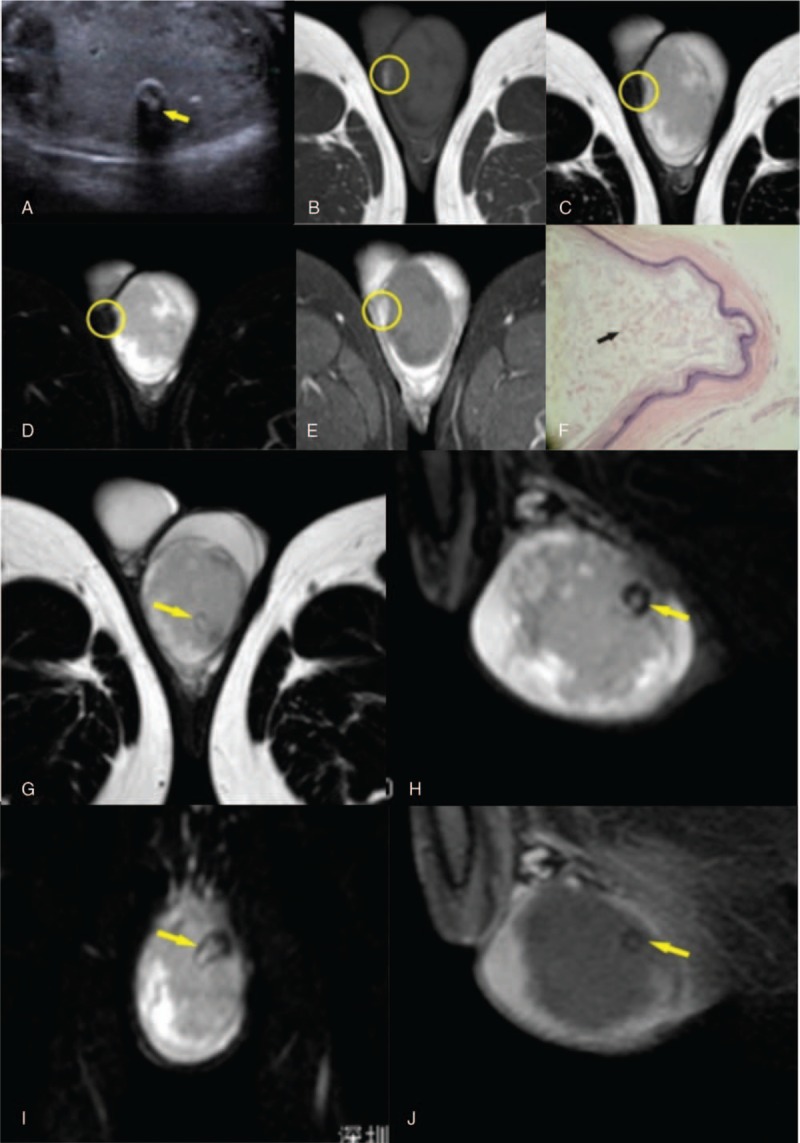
Twenty-six-year-old male who had a TECs with atypical characteristics on preoperative clinical examination and MR imaging. (A) Ultrasonography showing the lesion had a heterogeneous internal echotexture, with a central area of increased echogenicity accompanied by an acoustic shadow (yellow arrow). (B) T1-weighted images of the lesion showed a hyperintense nodule on the cyst wall (yellow circle). (C) T2-weighted images of the lesion showed a hypointense nodule on the cyst wall (yellow circle). (D) SPAIR images of the lesion showed a hypointense nodule on the cyst wall. (E) After Gd-DTPA injection, the nodule was enhanced on THRIVE images. (F) Pathology (HE × 20) showed a disorderly distribution of intracapsular keratides and no stratified structure (black arrow). (G–I) T2-weighted/SPAIR images displayed a small black ring sign. (J) After Gd-DTPA injection, the small black ring was not enhanced (yellow arrow) on THRIVE images.
